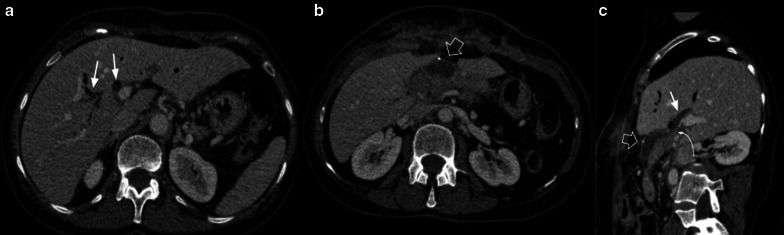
Biliary fistula 67-year-old female patient with increased fluid drainage after 60 days of pancreaticoduodenectomy due to ampuloma. (a, b, c) Axial and oblique sagittal portal venous phase CT scan. The intrahepatic biliary duct shows dilatation and fluid level within (white arrows in a and c). A collection in the surgical bed (empty arrow in b and c) and biliary connection (curved arrow in c) is also shown. Drainage analysis disclosed high bilirubin levels, which confirms the diagnosis of biliary fistula
